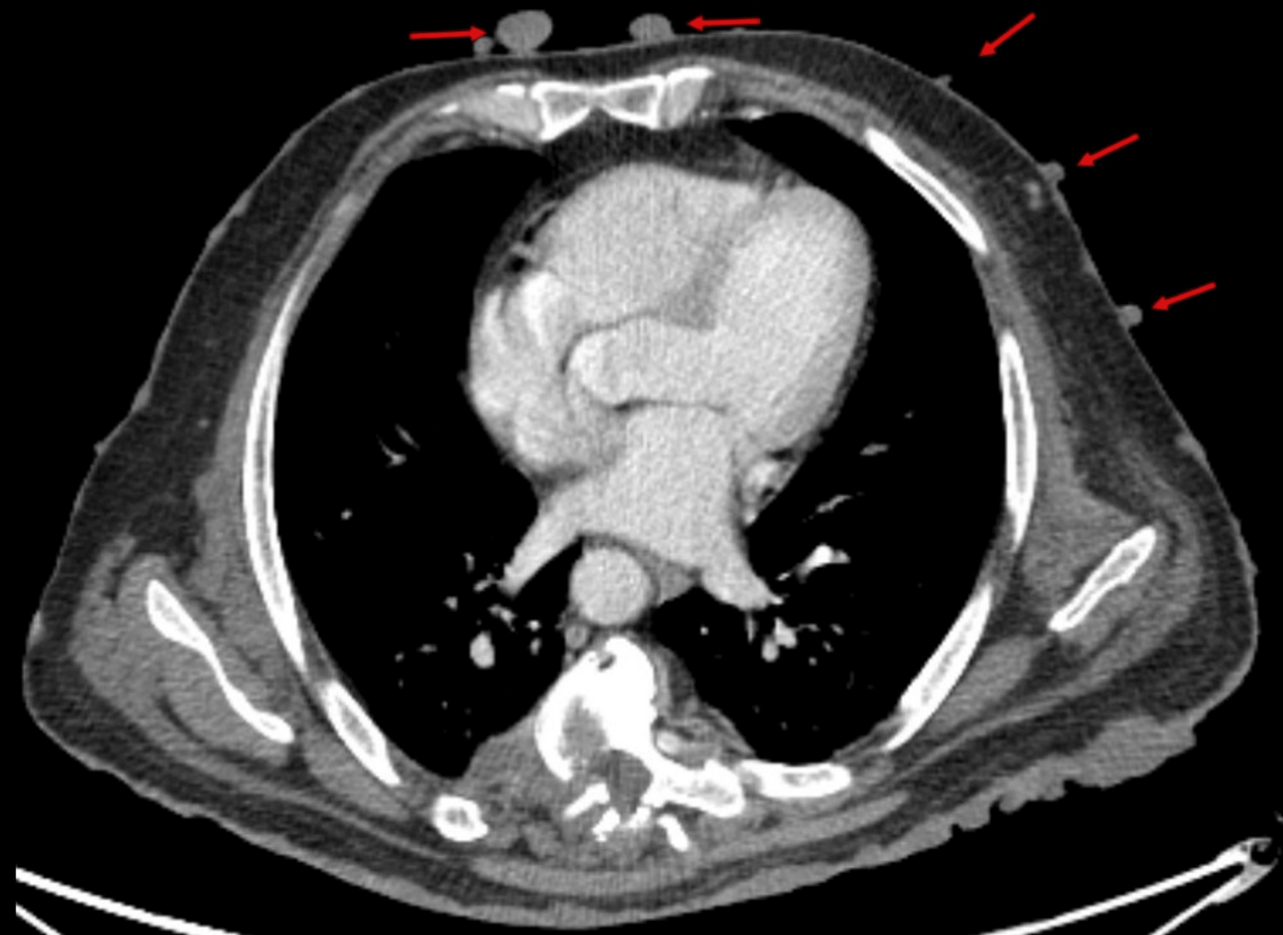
Axial sections of the contrast-enhanced computed tomography (CECT) chest in soft tissue window at the level of the heart showing multiple percutaneous enhancing soft tissue lesions (red arrows)
